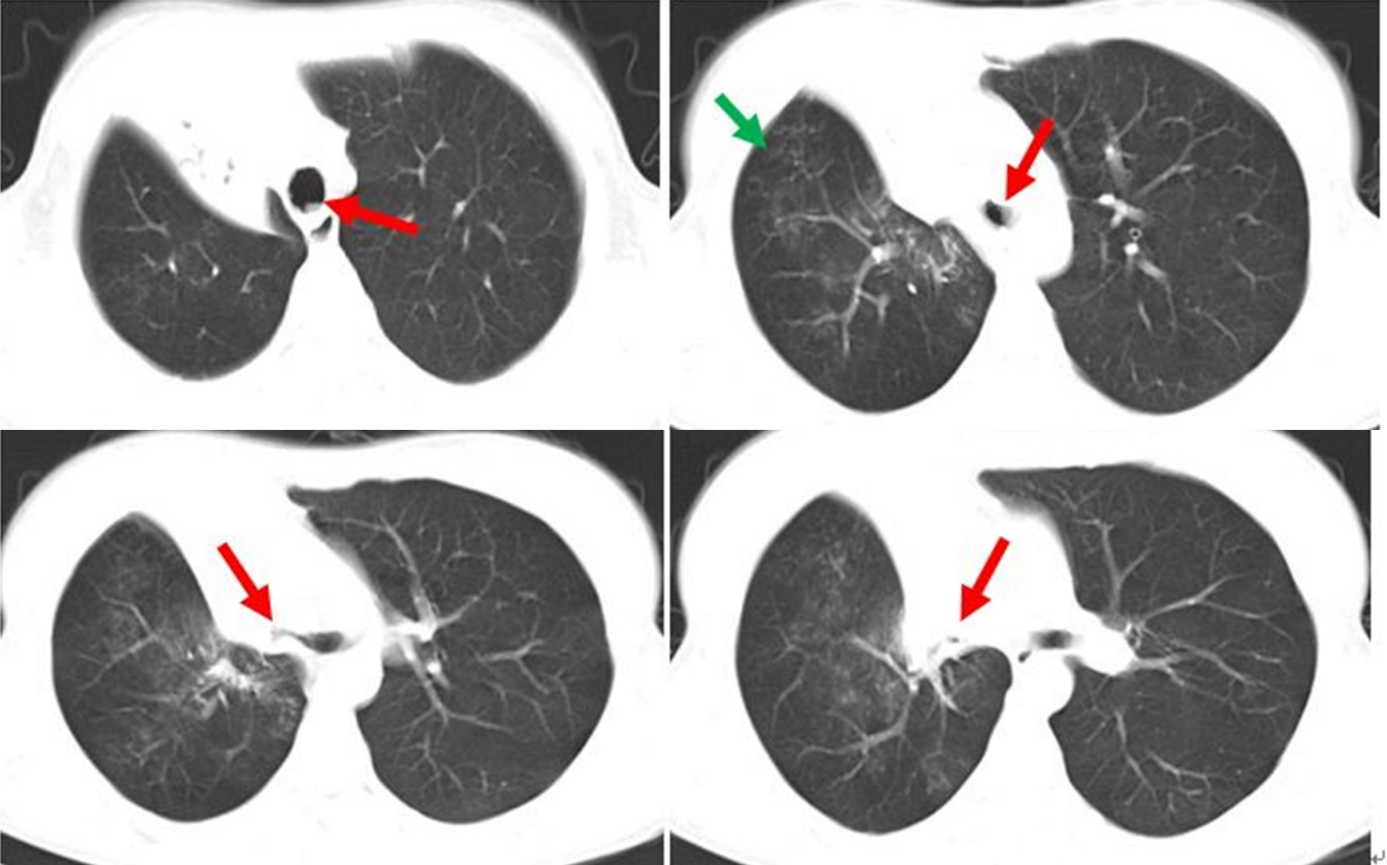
Computed tomography (CT) before treatment. CT scan: Lesions (green arrow) in the pulmonary parenchyma in the right lung; the distal portion of the trachea and the bronchial tree of the right lung were irregular and had varying degrees of stenosis (red arrow).
